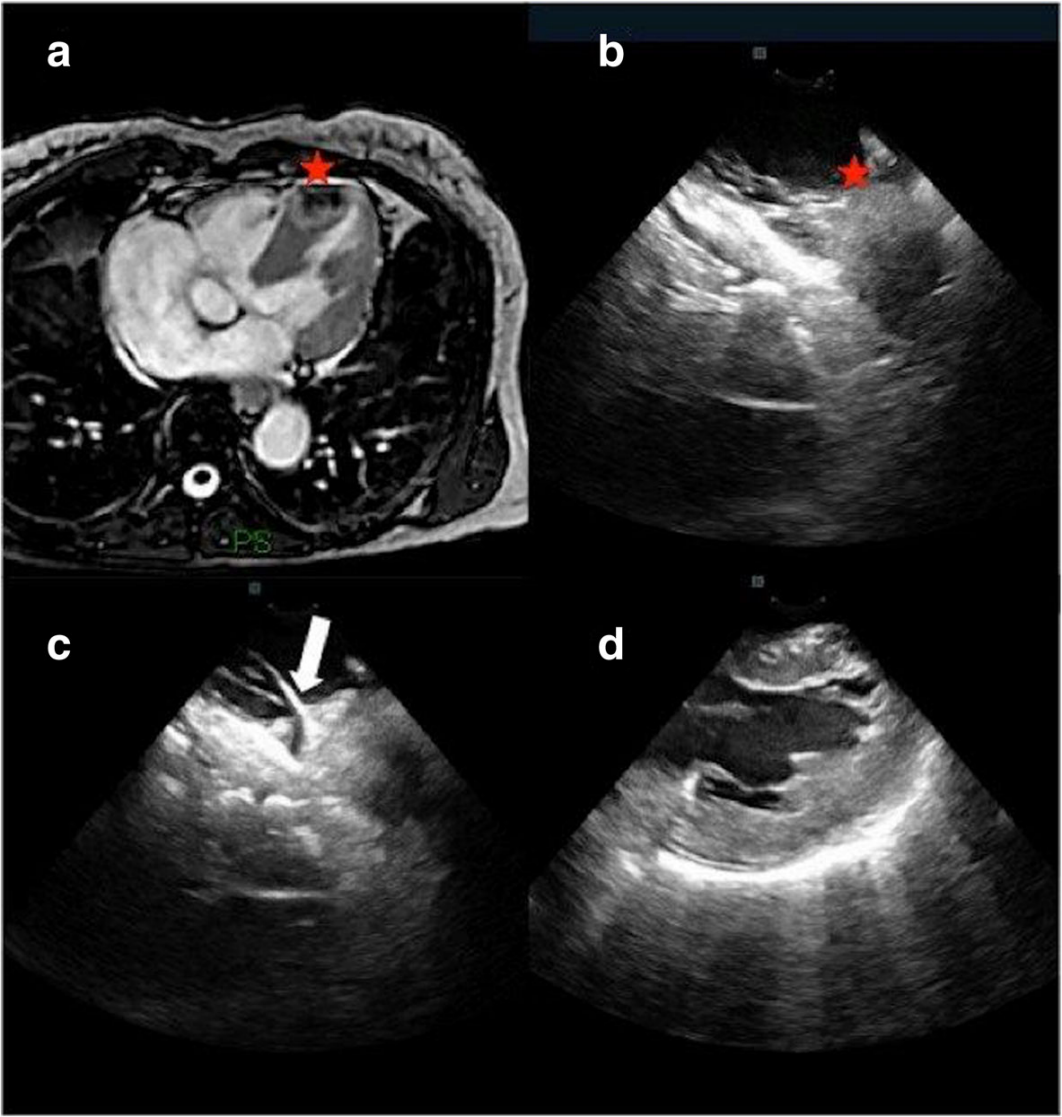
Cardiac magnetic resonance imaging: a 20 × 28 mm intramyocardial mass localized at the apex of the right ventricle (RV) is showed in panel (a). ICE shows the RV apical mass, panel (b). The bioptome (white arrow) is clearly visualized and is possible to confirm the good contact between the catheter and the mass, panel (c). After obtained all the biopsies needed, ICE is useful to exclude the presence of pericardial effusion, panel (d)
